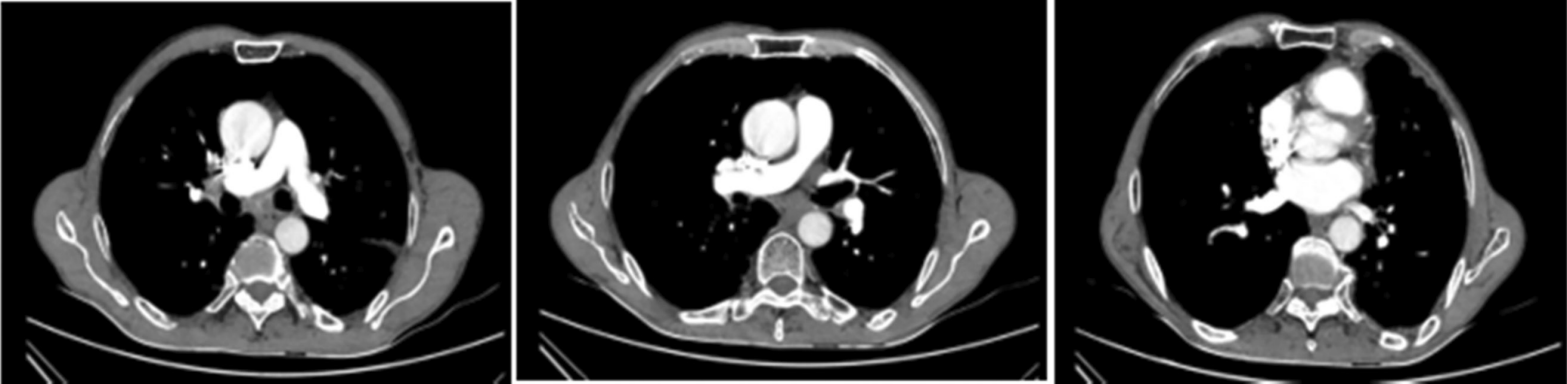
Lung computed tomography angiography (LCTA) revealed no sign of PTE.
